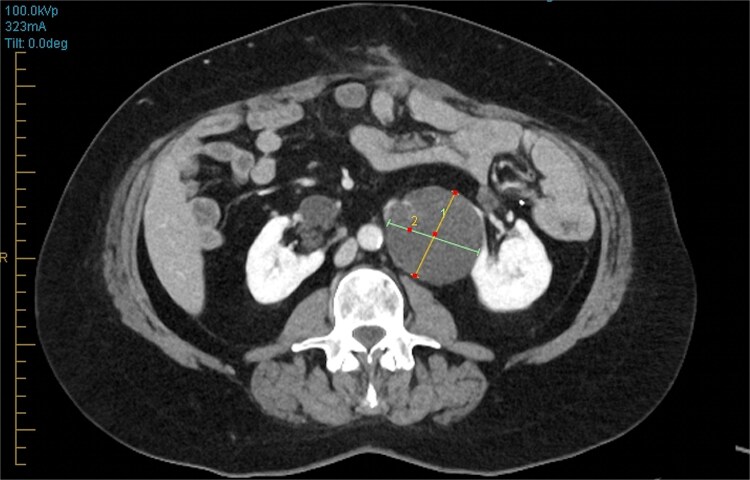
CT abdomen/pelvis axial slice showing complex left sided renal cyst.
